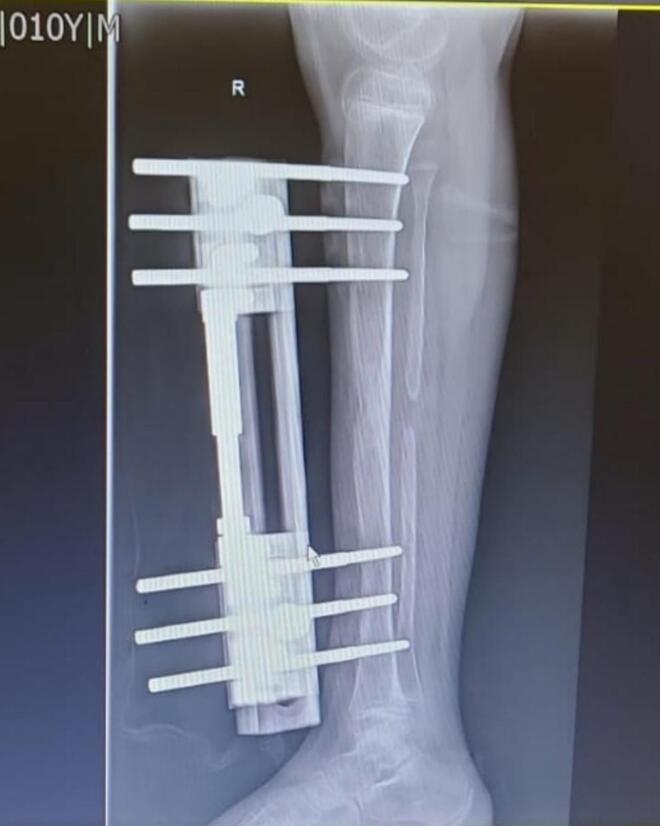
Xray Right Leg (Lateral view) taken just before implant removal confirming 3 cm increase in tibial length and clinical limb equalization.
